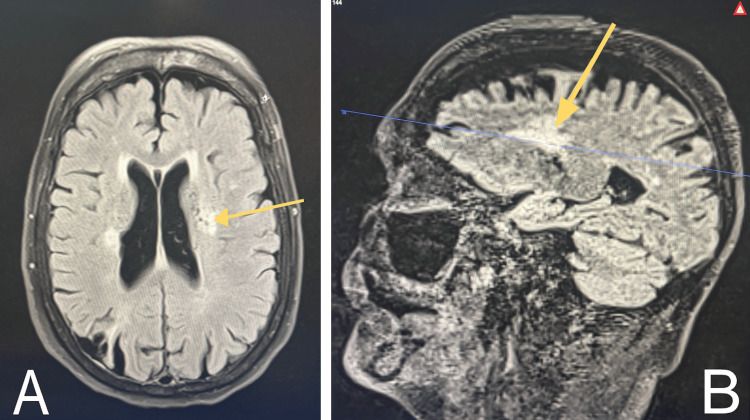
Noncontrast MRI of the brain showing extensive periventricular T2 FLAIR hyperintensities consistent with chronic demyelination (yellow arrows display hyperintensities). (A) Axial view. (B) Sagittal view FLAIR: fluid-attenuated inversion recovery
